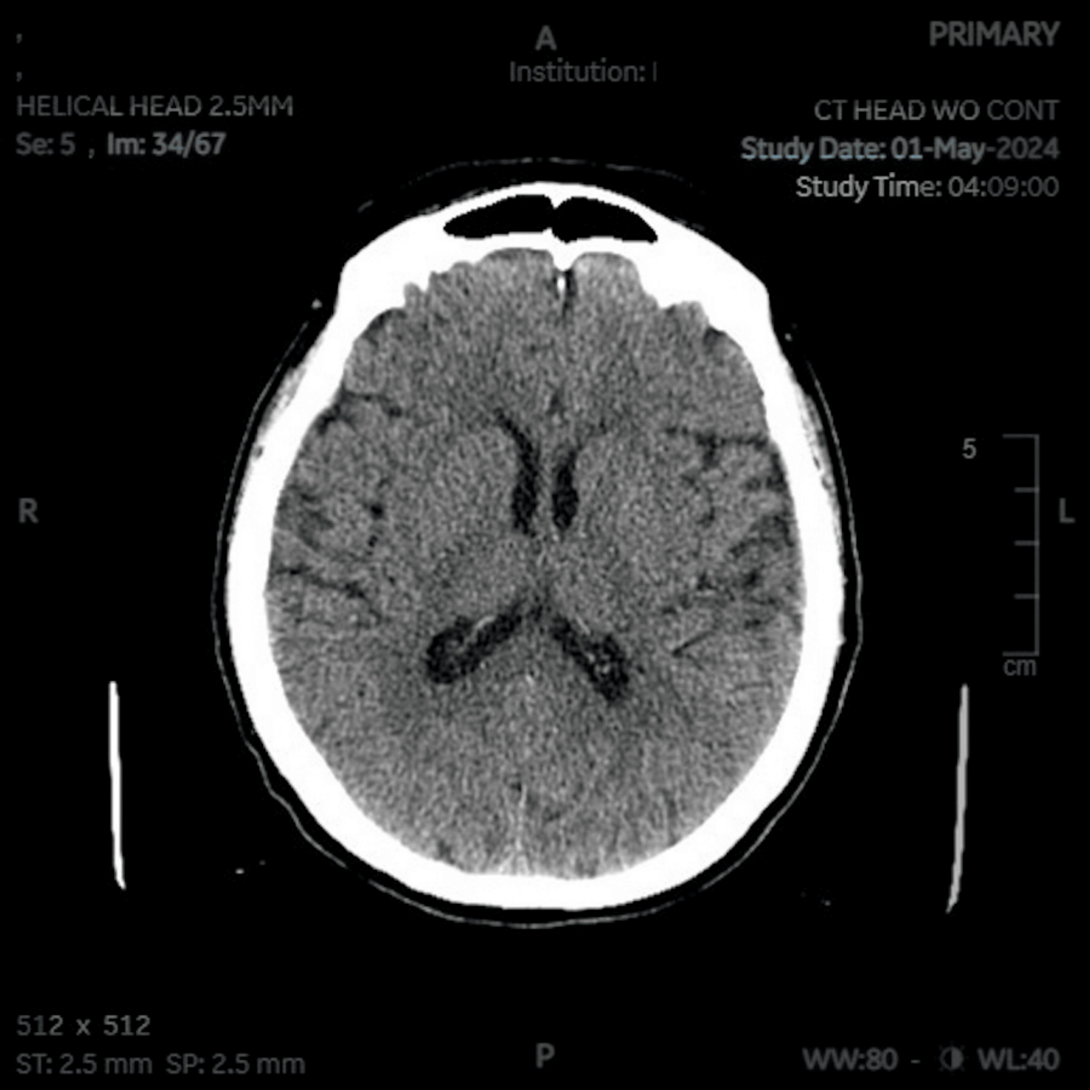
CT of the head with axial view demonstrating no subdural hematoma
